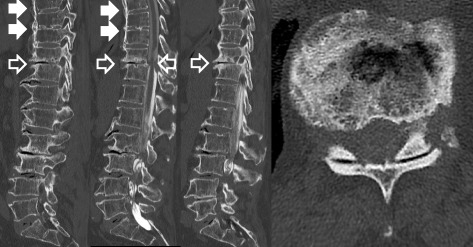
Myelogram-CT revealed T10 to T11 anterior spondylolisthesis and severe cord compression at T10/11 level (*white outline arrows*). Ossification of the posterior longitudinal ligaments and ossification of the ligamentum flavum were not seen at the T10/11 level. DISH was seen above the T10 level (*white solid arrows*)
